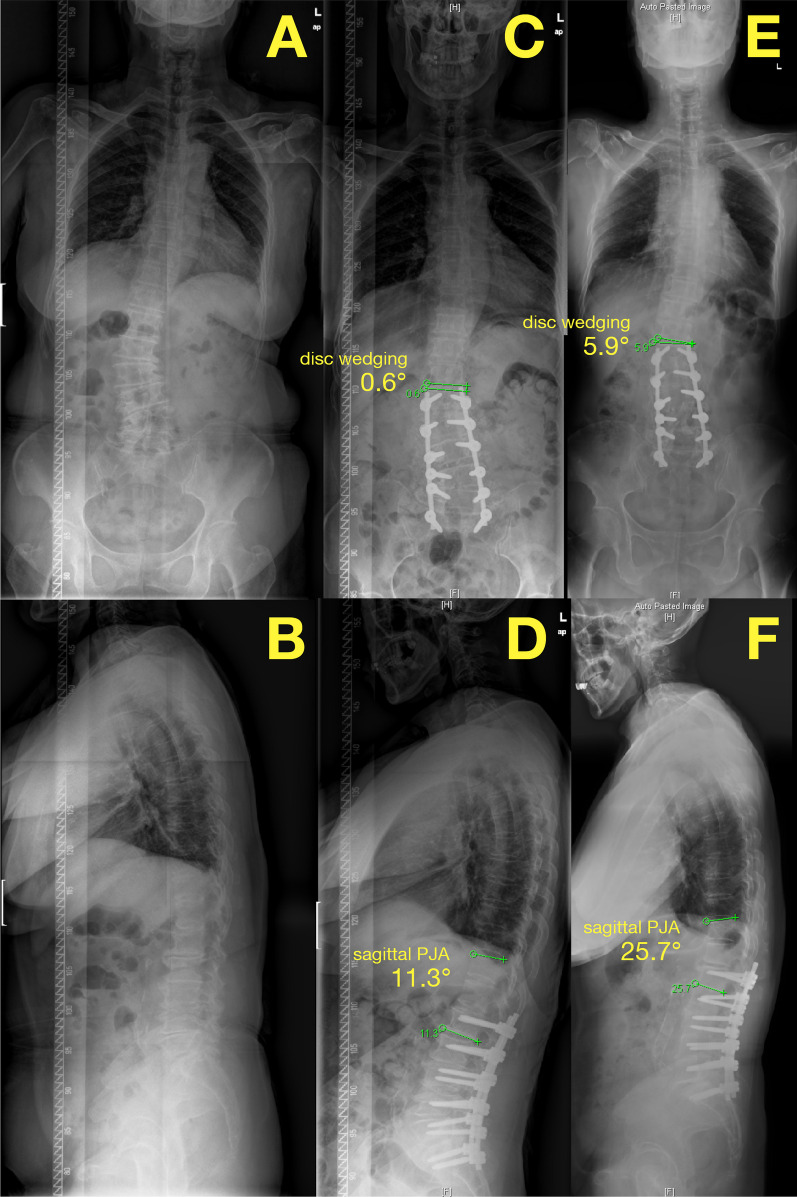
The preoperative (a, b), postoperative (c, d), and 2-year follow-up (e, f) X-ray plain films of a dF > 5 patient with UIV > FCRV (FCRV = T11, UIV = L1). The patient had pASD of both PCD (c, e, progression of disc wedging of 5.3°) and PJK (d, f, progression of sagittal PJA of 14.4°)
